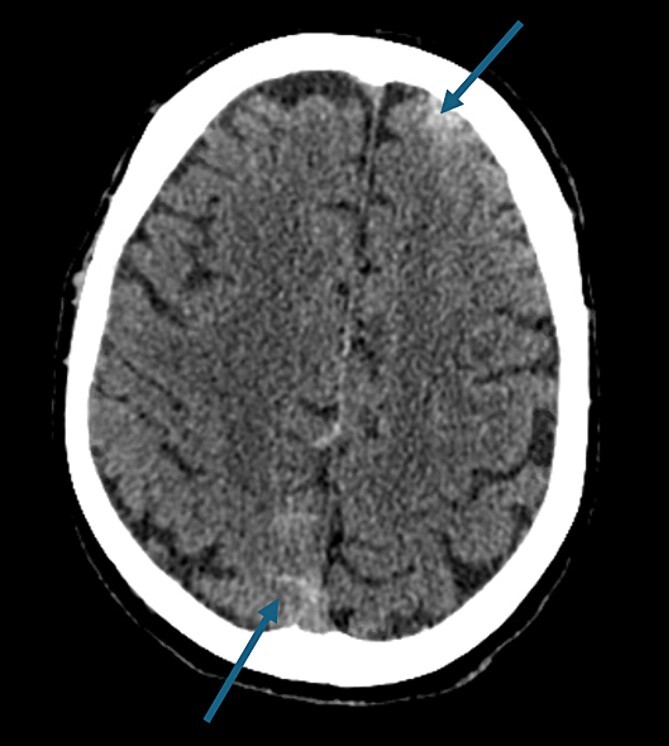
Non-contrast axial head computed tomography scan demonstrating biconvexity subarachnoid hemorrhage.
